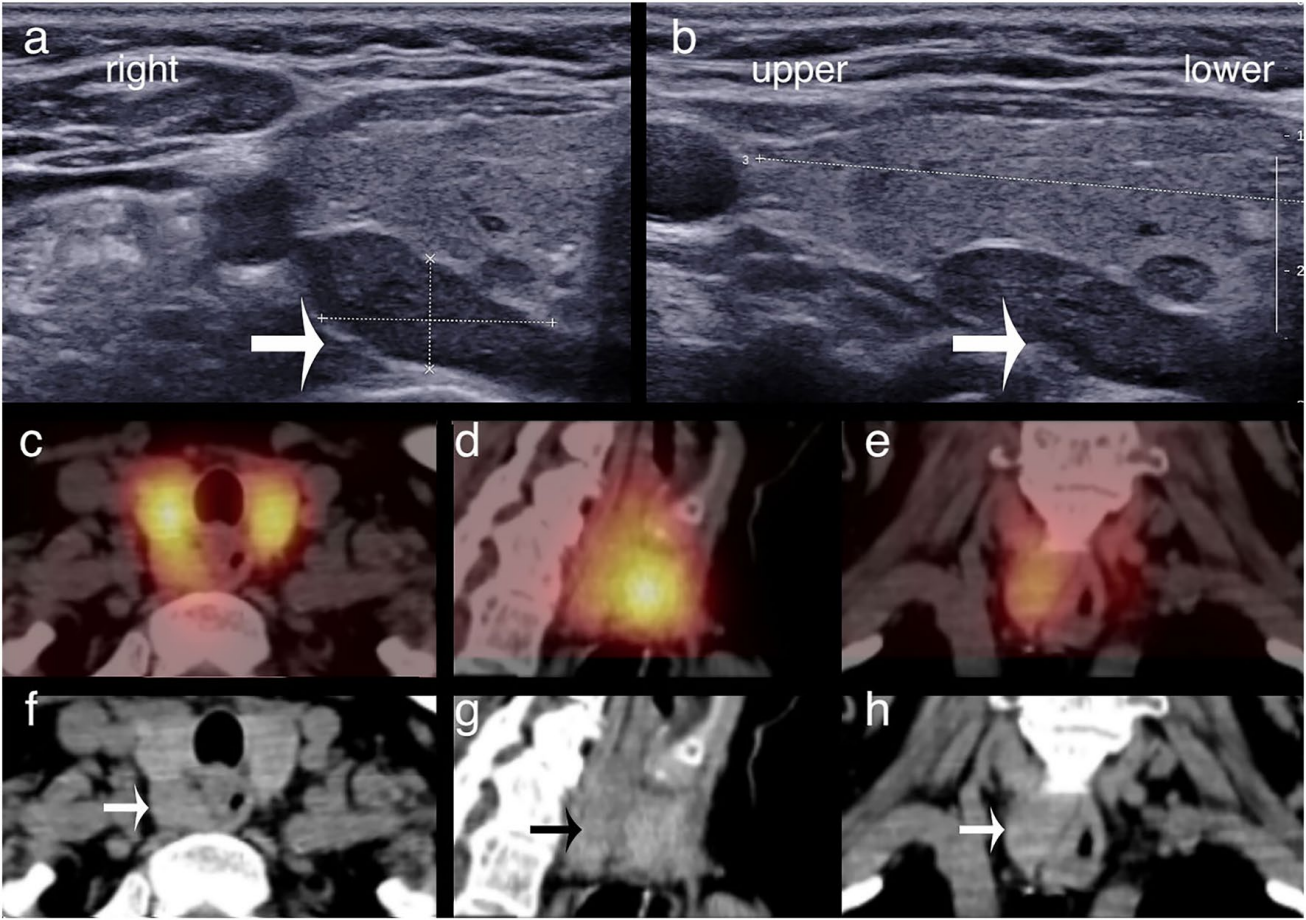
Upper parathyroid adenomas correctly localised by ultrasound and [^99m^Tc]pertechnetate/MIBI SPECT/CT. (a,b) Transverse and longitudinal ultrasound sections. (c–e) Fused [^99m^Tc]pertechnetate/MIBI SPECT/CT axial, sagittal and coronal slices and (f–h) present the corresponding CT slices all in the same patient with an upper right adenoma. Both ultrasound and [^99m^Tc]pertechnetate/MIBI SPECT/CT correctly identified the adenoma (arrows on ultrasound and CT images) as an upper adenoma, adjoining the middle third of the thyroid lobe.
